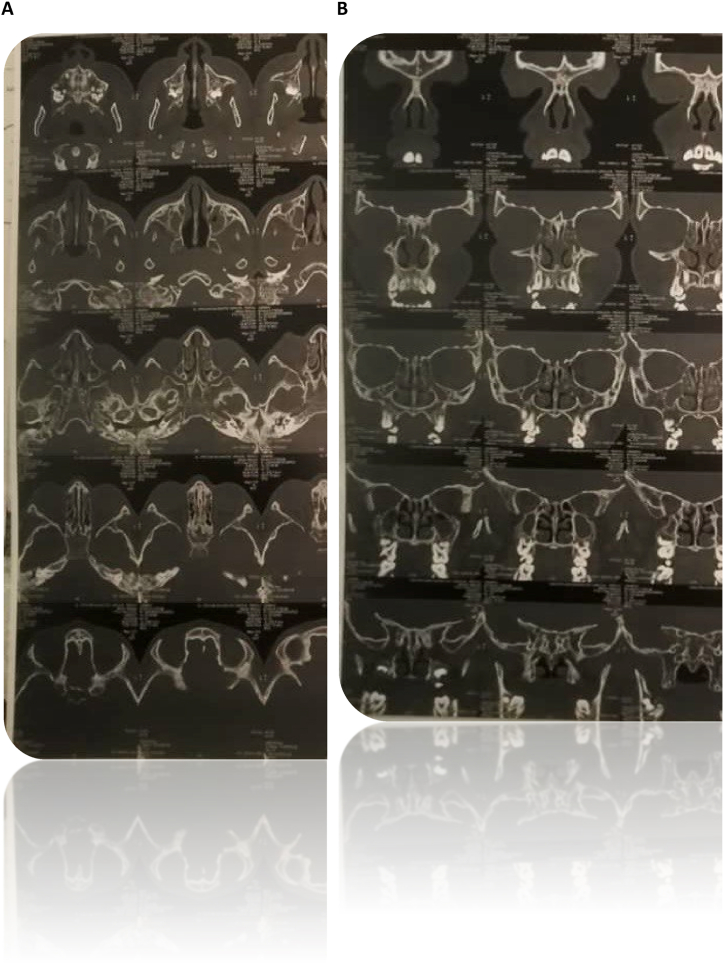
Ct scan of the paranasal sinuses (Axial and coronal views) Plain CT Scan for the Evaluation of Sinus Pathology. In the review of the CT scan of the paranasal sinuses, there is near-total opacification of the maxillary and sphenoidal sinuses, as well as ethmoidal air cells. Notable mucosal thickening and bony border irregularities of the paranasal sinuses are also observed. There is associated opacification of the left middle ear cleft suggestive of otitis media. These radiological features are consistent with chronic pansinusitis. Due to the history of recurrent chest infections and chronic sinusitis, further evaluation to rule out Primary Ciliary Dyskinesia (Immotile Cilia Syndrome) is recommended.
